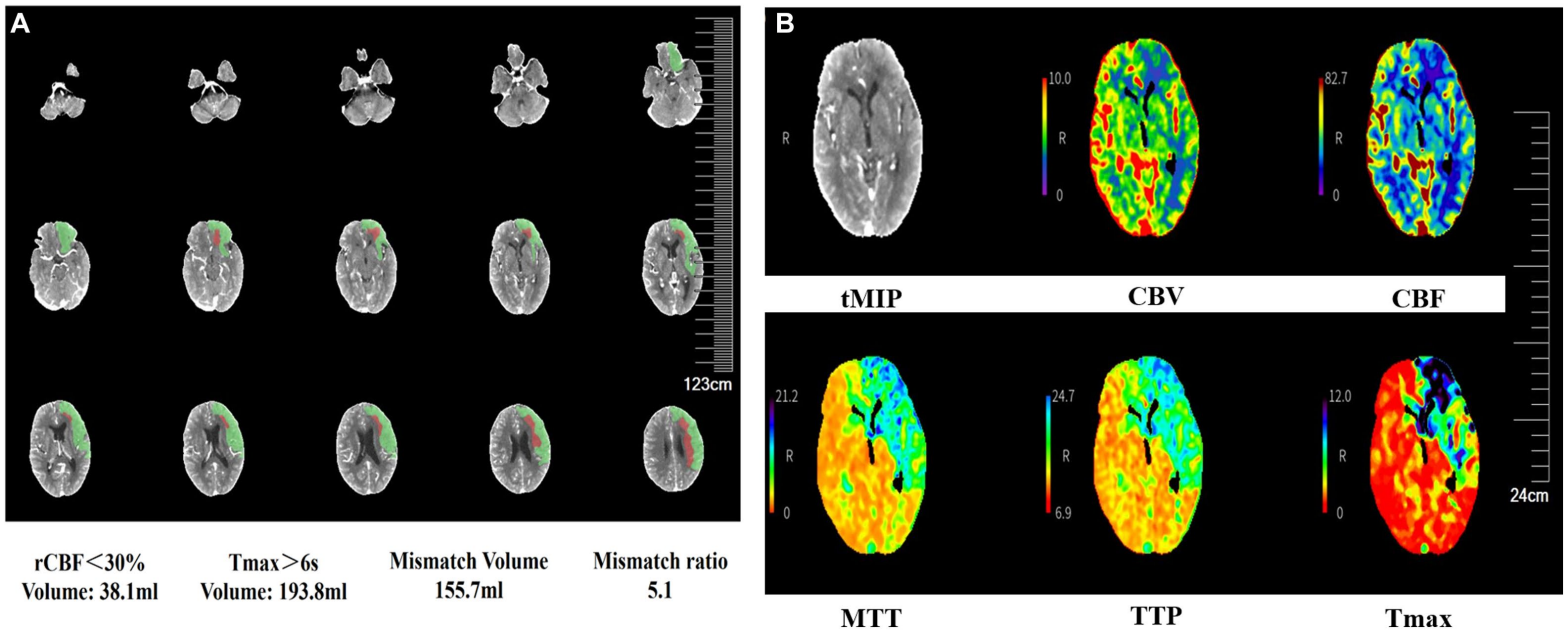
CTP images show cerebral blood flow following carotid stent thrombosis. CTP shows the ischemic penumbra after acute ischemia (A). CBF and CBV decrease, and TTP and MTT increase in the left cerebral hemisphere, indicating left cerebral ischemia (B). CTP, computed tomography perfusion; CBF, cerebral blood flow; CBV, cerebral blood volume; TTP, time-to-peak; MTT, mean transit time; rCBF, relative cerebral blood flow; Tmax, time to top; tMIP, temporal maximum intensity projection.
